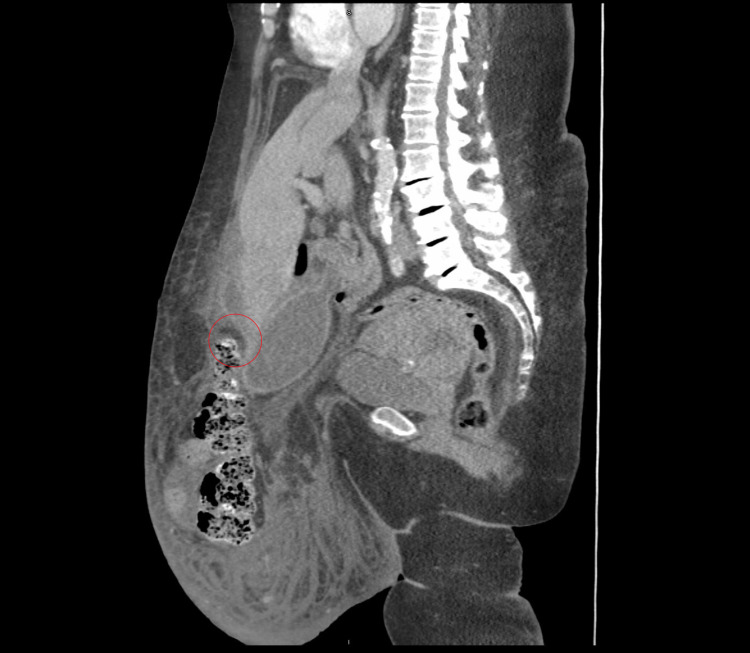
Sagittal CT of the abdomen and pelvis. Sagittal reconstruction of CT abdomen and pelvis done on hospital day 1 demonstrates rectus diastasis with a large hernia consistent of omentum, large and small intestine, right hepatic lobe of the liver, gallbladder, and urinary bladder (not shown). There is ongoing gallbladder distension and thickening. Pericholecystic loculated rim enhancing fluid (red circle) is seen on the anterior inferior right hepatic lobe.
